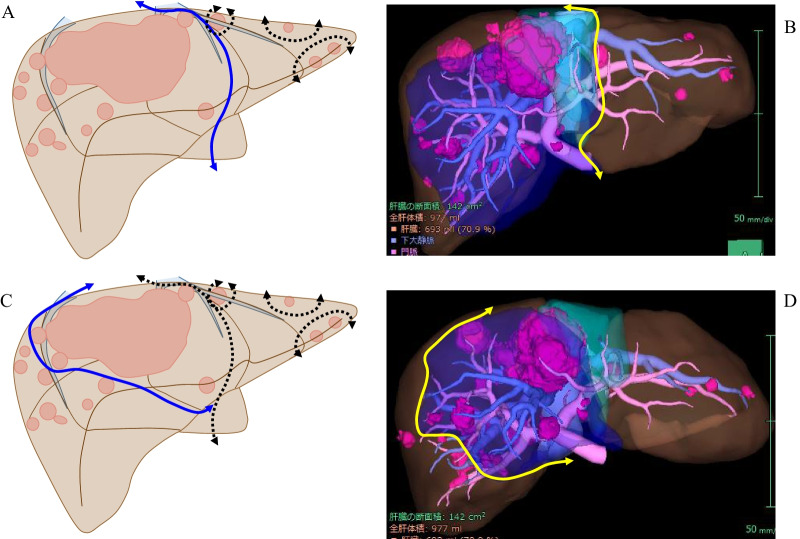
ALPPS procedure in the present patient. Panel a shows the scheme of parenchymal division at the umbilical fissure (blue line) following 3 partial resections within the left lateral section (black dotted line). Panel b shows territories of S4 portal pedicles (green area) and MHV (blue area) as depicted by 3-dimensional (3D) computed tomography. The resection line at the left umbilical fissure is shown in yellow. Panel c shows an additional line of resection to excise the territories of both S4 and the MHV (in blue). Dotted lines indicate 3 partial resections and the resection line at the umbilical fissure. Panel d shows a 3D-CT image with the line for additional resection of S4 and the MHV territory in yellow
